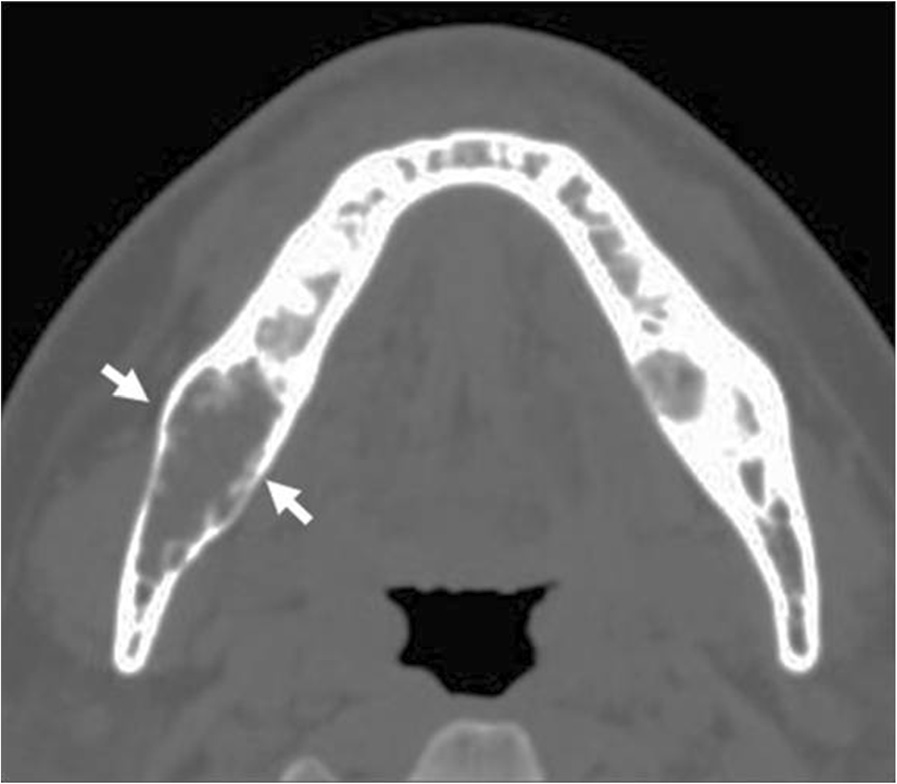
An axial computed tomography scan indicating a lesion on the right side of the mandible with thin residual bony trabeculae, and expansile invasion (arrows).
